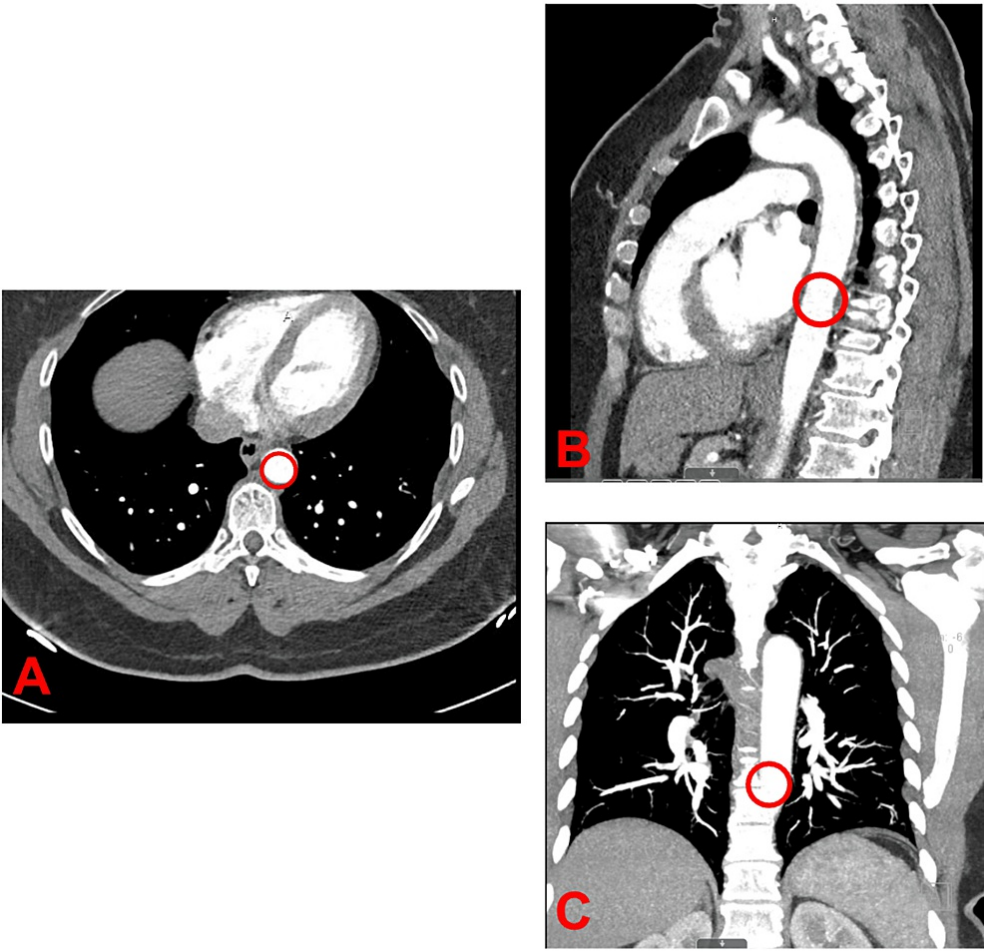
CTA of the chest post-anticoagulation treatment showing resolution of previously noted pedunculated aortic thrombus A. Axial view of CTA showing resolution of pedunculated thrombus. B. Sagittal view of CTA showing resolution of pedunculated thrombus. C. Coronal view of CTA showing resolution of pedunculated thrombus. The encircled region in A, B, and C indicates where the pedunculated thrombus was found in the descending thoracic aorta before treatment was initiated. CTA, computed tomography angiogram; AP, anteroposterior
